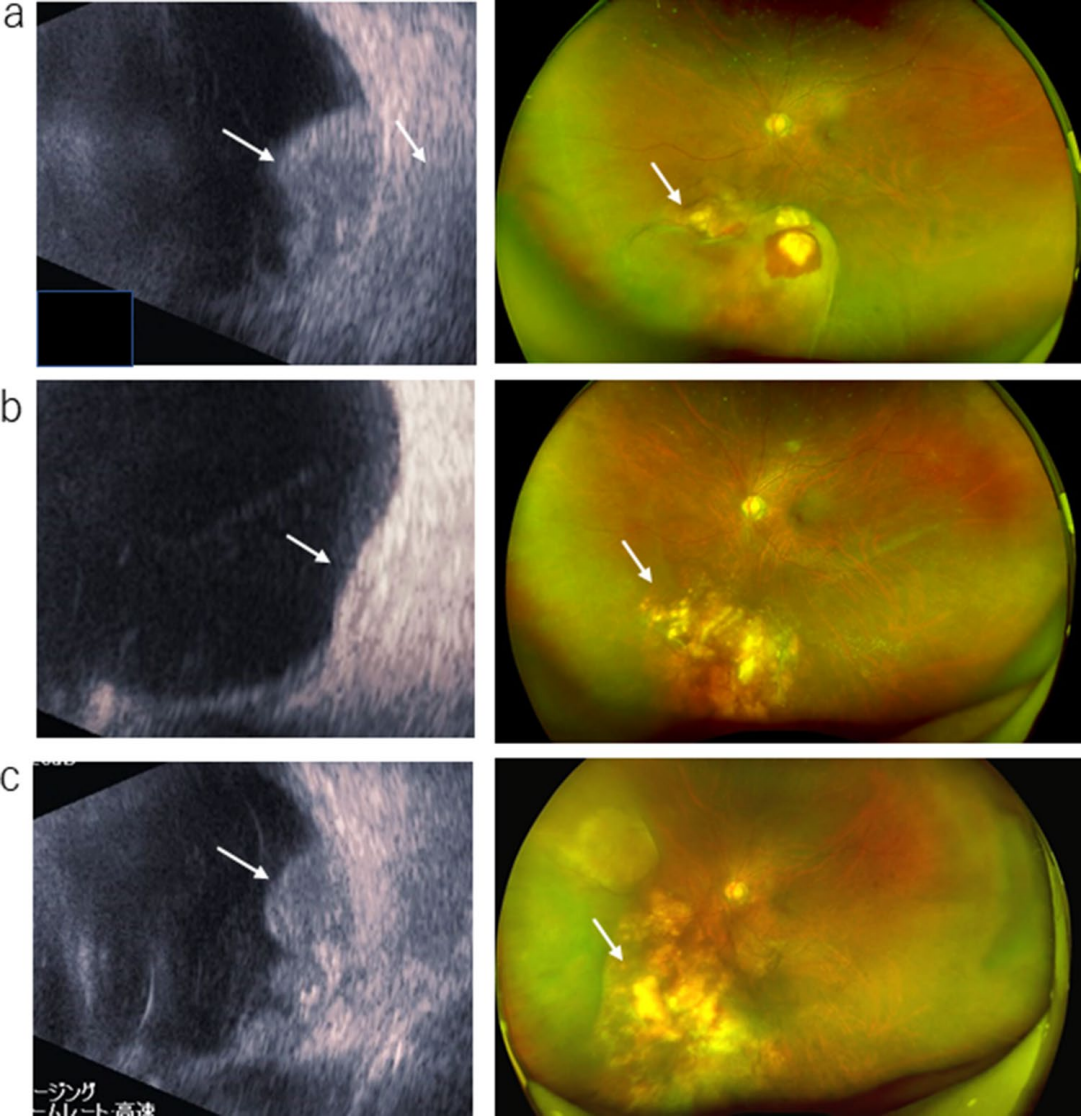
Fundus and echocardiographic findings a at initial ophthalmologic examination, b after radiotherapy, c before oophorectomy
